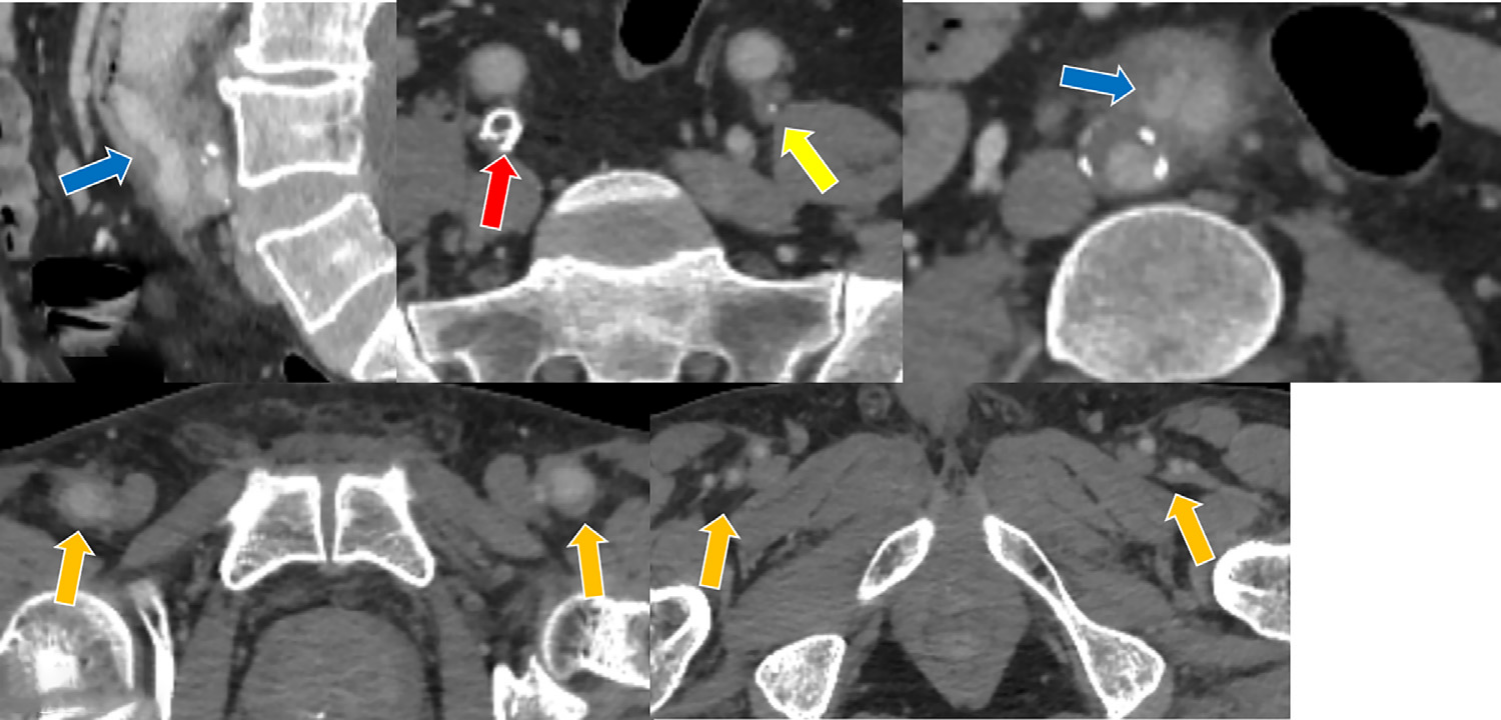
Computed tomography angiography (CTA) of a 47-year-old male demonstrating right common iliac artery occlusion (red arrow) and left external iliac artery occlusion (yellow arrow), both treated with a bilateral aortobifemoral bypass graft (green arrow). The image also shows migration of the graft into the duodenal lumen (purple arrow), erosion of the right graft limb (blue arrow), and patency of the bilateral distal graft anastomoses (orange arrow).
